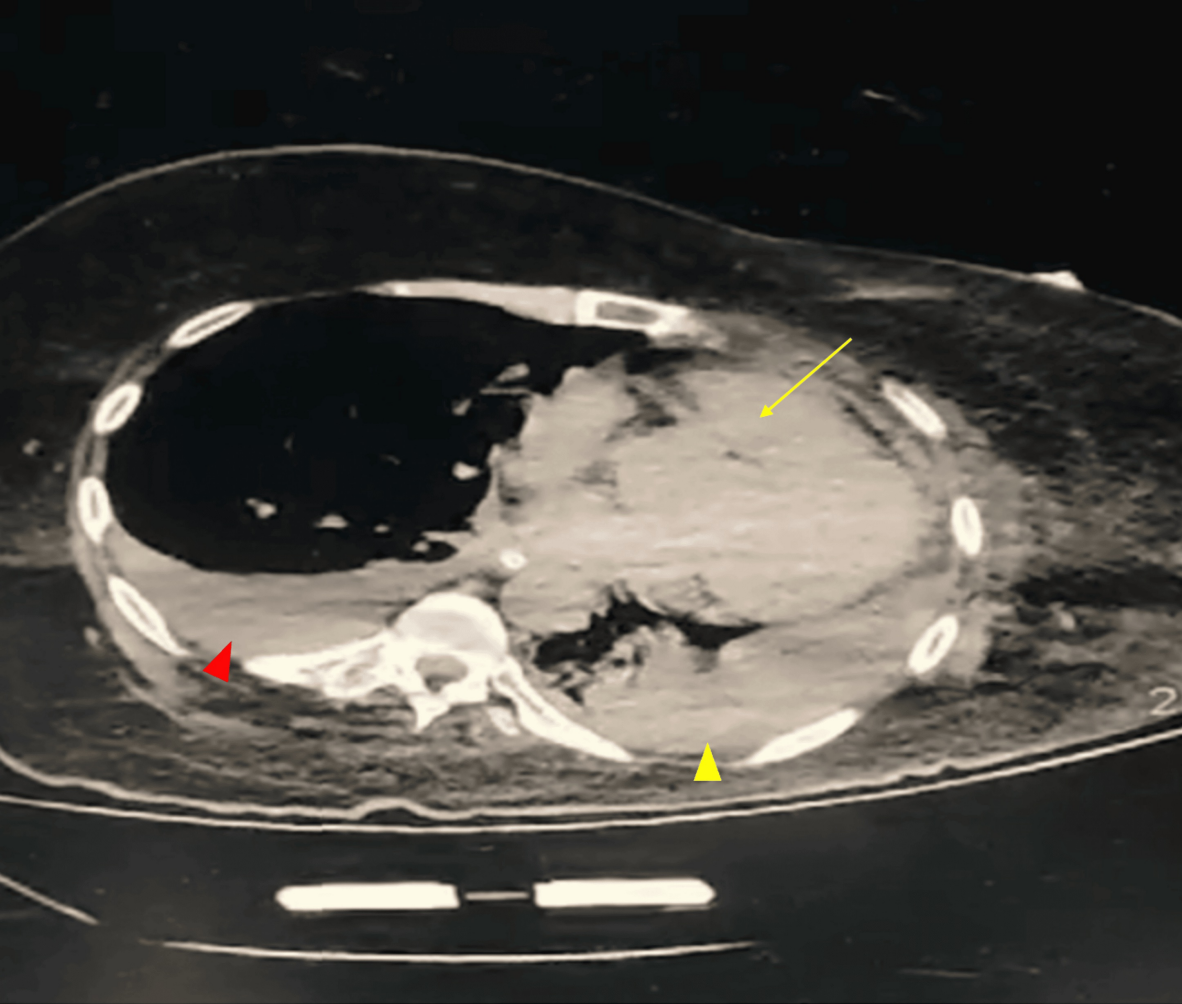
Non-contrast chest CT (axial view) performed 48 hours after admission, showing consolidative patches with air bronchograms in the left lung, relative left lung collapse with mediastinal shift to the left, bilateral basal atelectatic bands, and resolved air embolism (yellow arrow). Additionally, bilateral pleural effusions are present: mild on the right (red arrowhead) and moderate, encysted on the left (yellow arrowhead).
